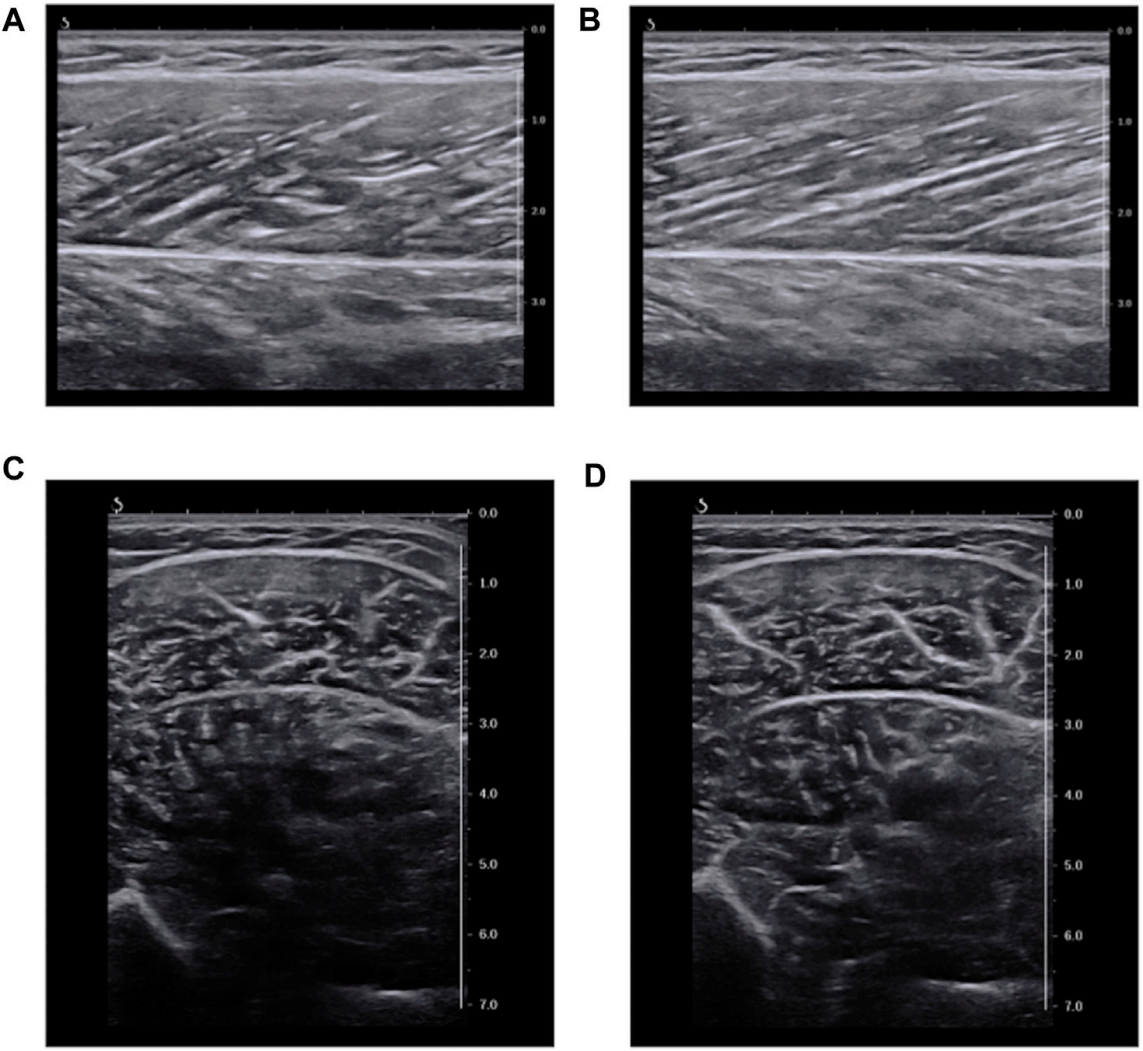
Representative ultrasound B-mode image. (A): longitudinal images at 20° ankle plantar-flexion. (B): longitudinal images at 20° ankle dorsiflexion. (C): transverse images at 20° ankle plantar-flexion. (D): transverse images at 20° ankle dorsiflexion. The values of echo intensity calculated using a region of interest (RoI) which included as much of the muscle as possible while avoiding fasciae and bone (maximum RoI) were (A) 109.3 a.u., (B) 118.4 a.u., (C) 76.0 a.u., and (D) 77.8 a.u.
